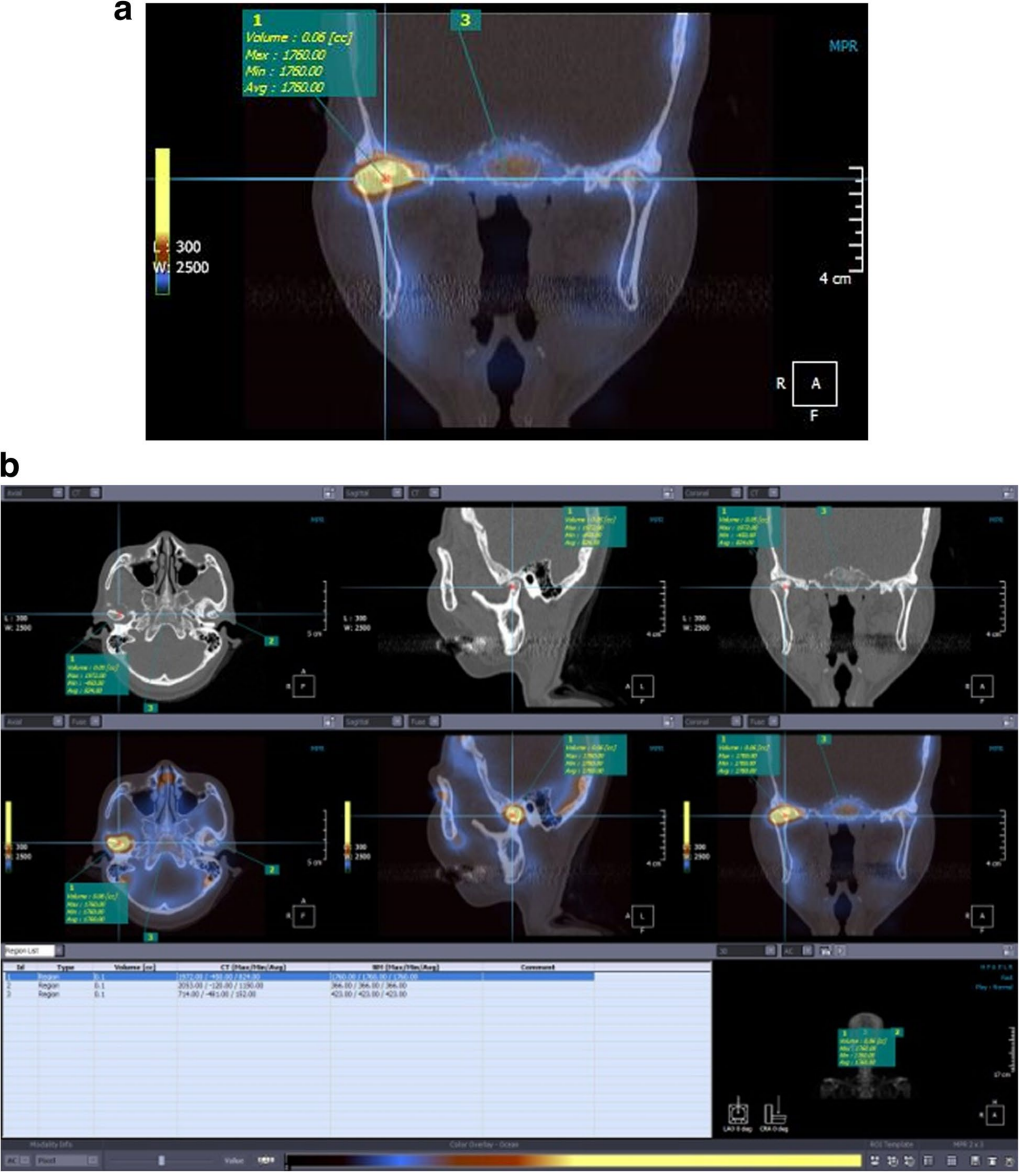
Region of interest (ROI) drawn over the right condyle to calculate the maximum, minimum, and average radiotracer uptake count on bone SPECT and facial CT-fused image. a Radiotracer count was calculated by ROI (3.0 × 3.0 × 3.0 pixel) on coronal image. b Radiotracer count was calculated by ROI (3.0 × 3.0 × 3.0 pixel) on 3 planes (axial, sagittal, coronal view). Right, left condyle, and clivus also calculated for the ratio in each subject
